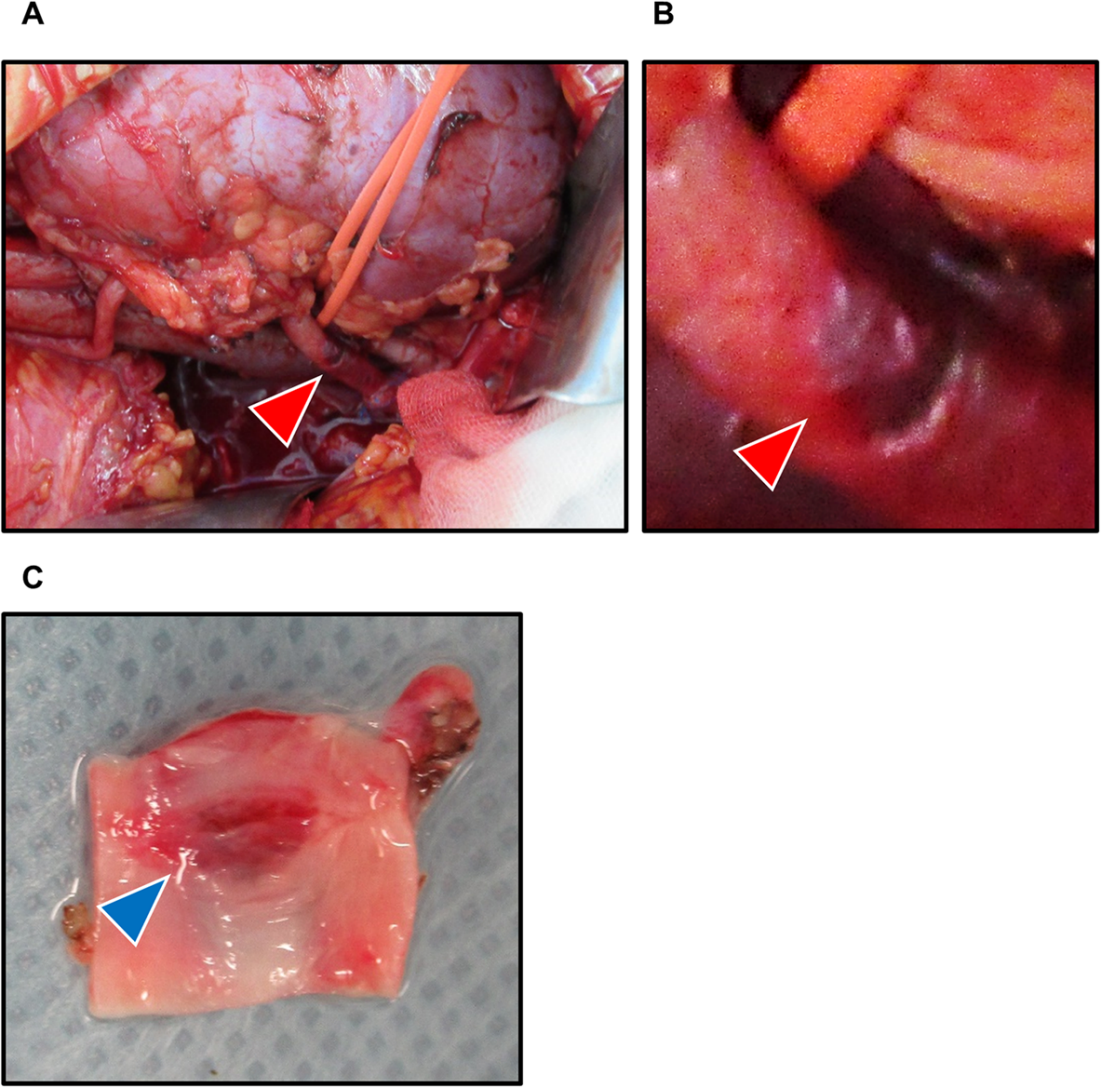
Representative macroscopic images of the allograft and injured transplant kidney artery. Immediately after vascular anastomosis (internal iliac artery-renal artery, external iliac vein-renal vein), Doppler ultrasonography shows an increase in the systolic blood velocity; thus, arterial anastomotic stenosis was suspected and explored. As a result, a color change is observed at the transplanted renal artery (red arrow). The cause of transplant renal artery stenosis was artery dissection (a whole image; b magnified image). Resected transplant renal artery was cut vertically, and artery dissection was observed macroscopically (blue arrow: c)
